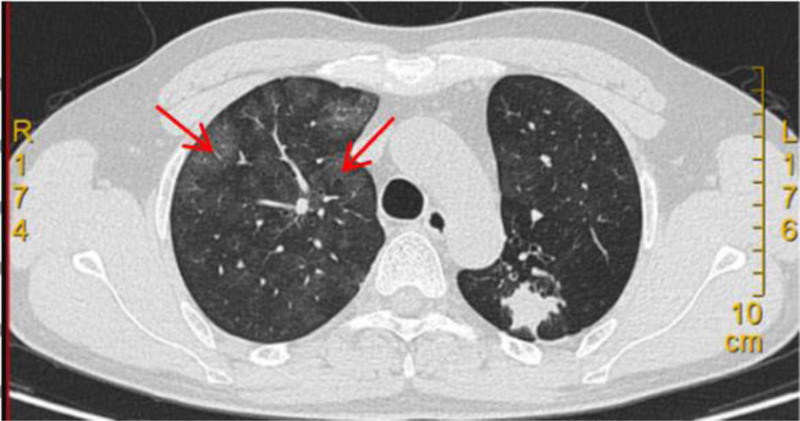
Chest CT scan on September 26, 2023 bilateral diffuse ground-glass opacities (red arrow). CT = computed tomography.
